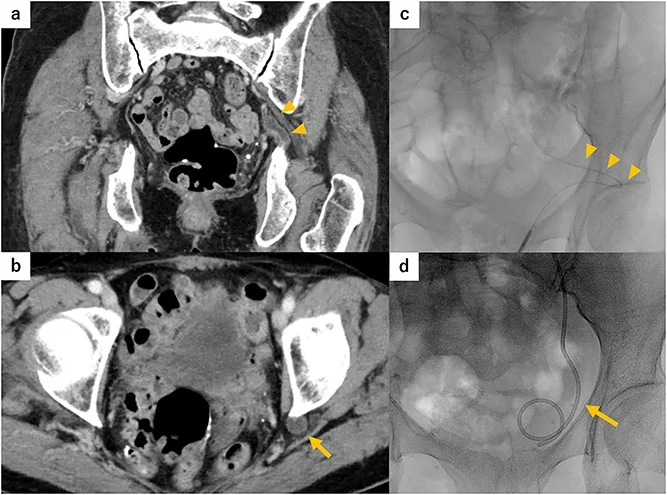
Preoperative imaging and ureteral stenting; (a) contrast-enhanced abdominal CT (coronal view) demonstrating herniation of the left ureter into the sciatic foramen (arrowhead); (b) contrast-enhanced abdominal CT (axial view) showing the left ureter herniating into the sciatic foramen (arrow); (c) fluoroscopic image obtained during insertion of the left ureteral catheter, confirming herniation of the ureter into the sciatic foramen (arrowhead); (d) fluoroscopic image after placement of the left ureteral catheter; the ureteral catheter (arrow) is correctly positioned, with resolution of the herniation.
